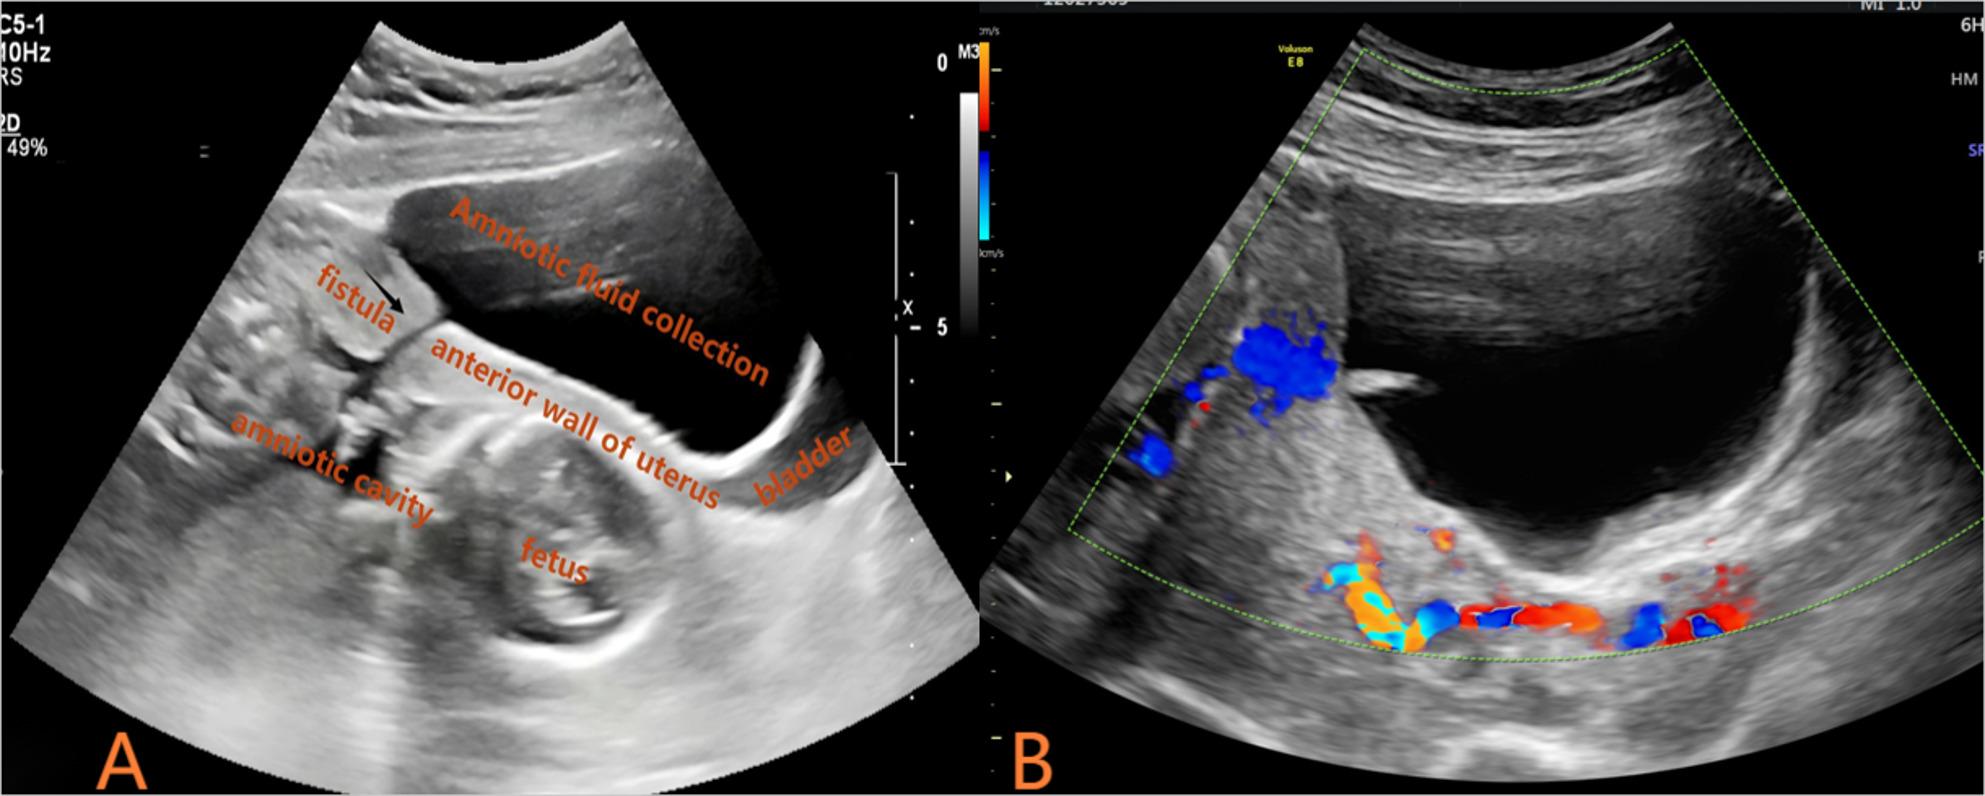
Transabdominal ultrasound at 19 weeks and 6 days of gestation. A sagittal view of the lower anterior uterine wall shows a slit-like defect (arrow) at the previous fetoscopic puncture site, with a large anechoic fluid collection within the vesicouterine pouch. B No vascular flow was detected within the collection on color Doppler imaging
